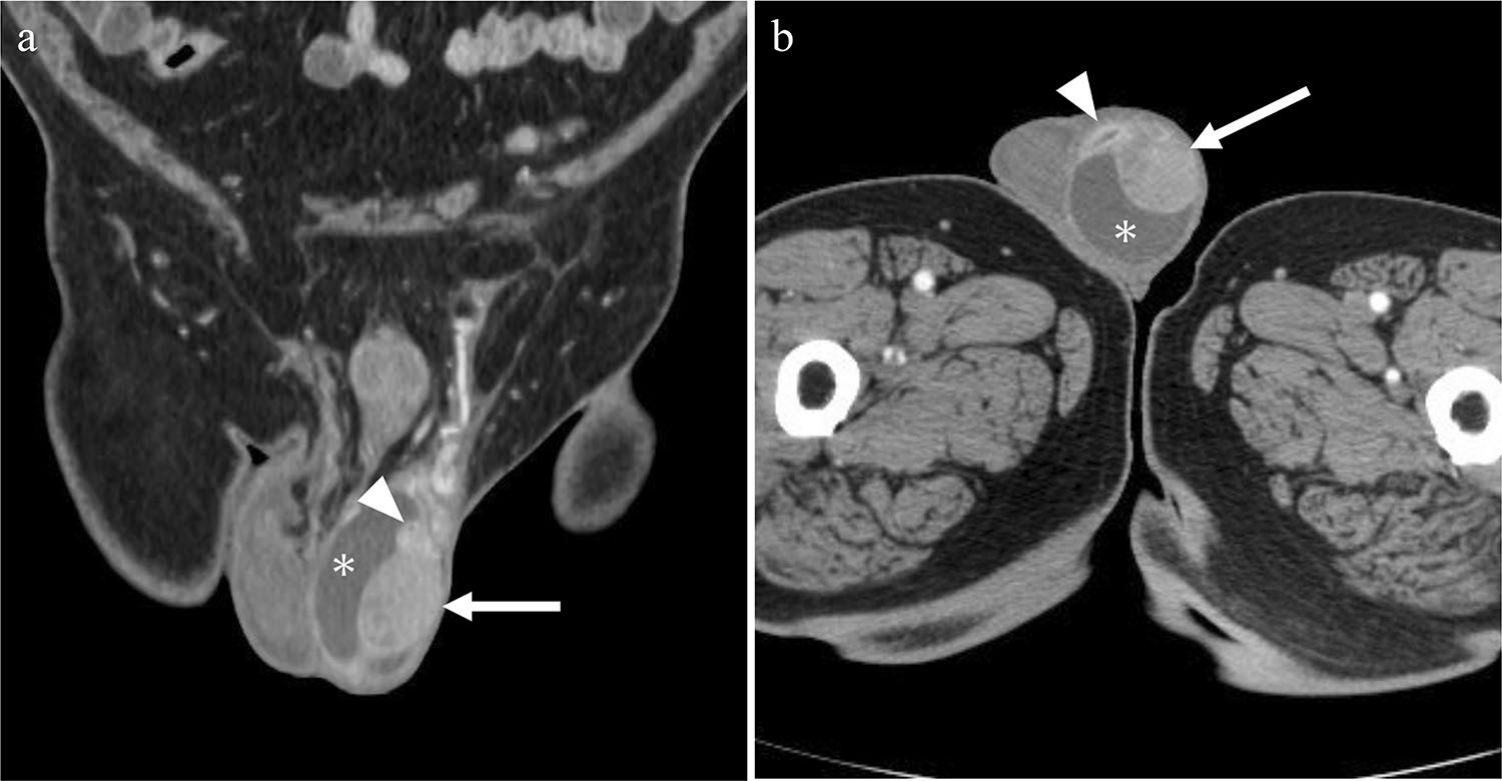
Man in his 70 s with fever who tested positive for Gram-positive bacilli. a Coronal contrast-enhanced CT shows enlargement and strong enhancement of the left epididymis (arrowhead) and testis (arrow), accompanied by thickening of the scrotal wall and reactive hydrocele (asterisk). These findings are indicative of epididymo-orchitis. b The axial plane reveals an abscess formation (arrowhead) is seen in contact with the testis (arrow) and hydrocele (asterisk)
